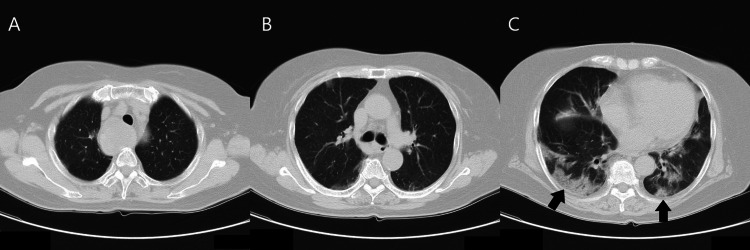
(A-C) Chest computed tomography images taken five days before being transferred to our hospital Peripheral ground-glass opacities are evident, predominantly in both lower lobes of the lungs.
